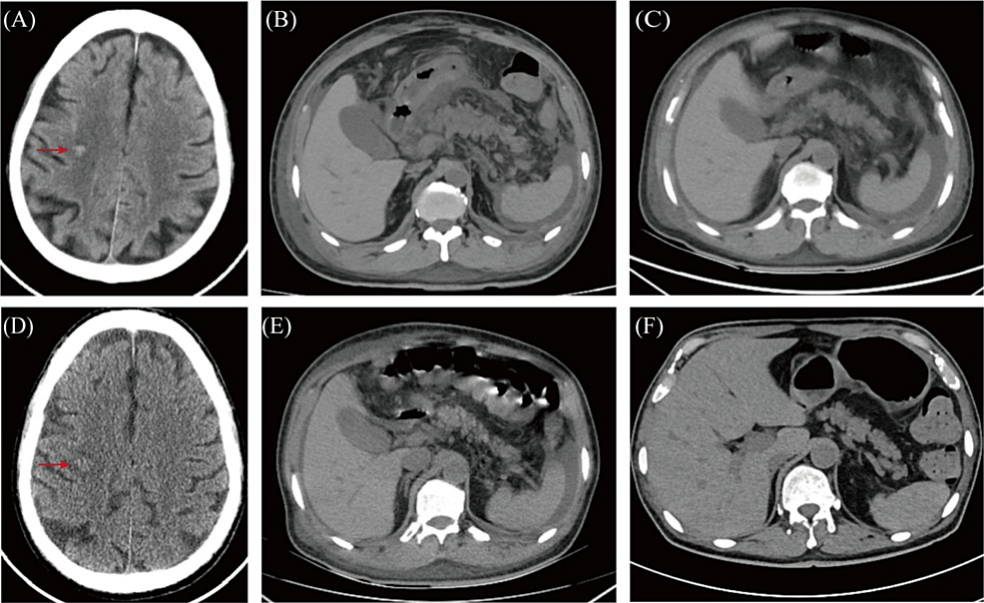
(A) The cranial CT on D1 showed a hemorrhagic focus in the right centrum semiovale; (B) the abdominal CT at night on D8 showed abdominal effusion and subcutaneous exudation; (C) the abdominal CT in the afternoon on D9 showed abdominal effusion, pancreatic edema, and subcutaneous effusion; (D) the cranial CT on D13 showed that the hemorrhagic focus in the right semiovular centrum had become less dense and the peripheral edema had increased; (E) the abdominal CT on D13 showed that the peripancreatic fat space was blurred, and the seroperitoneum and peripancreatic exudate were slightly resorbed; (F) the abdominal CT on D28 showed that the peripancreatic exudate had been resorbed and the seroperitoneum had decreased.
